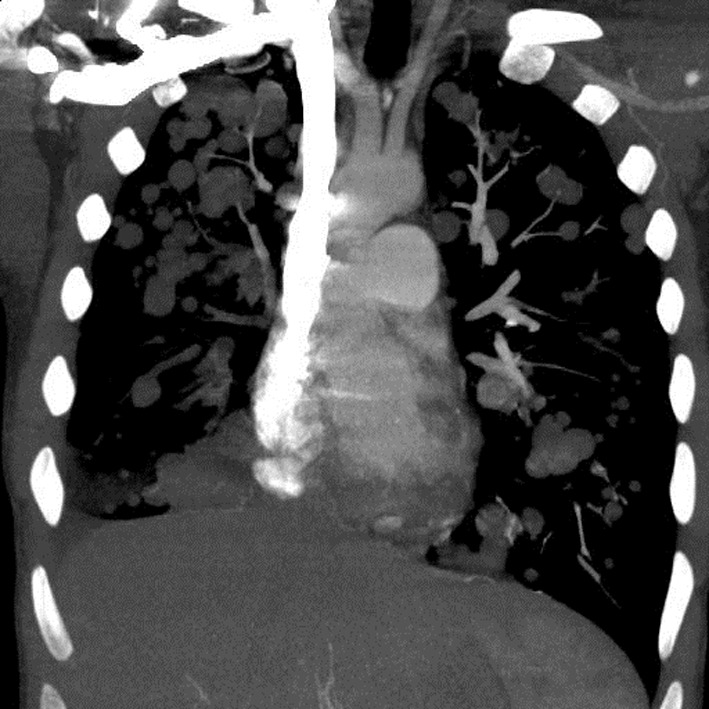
Coronal section of CT chest showing bilateral cannonball pulmonary nodules.
